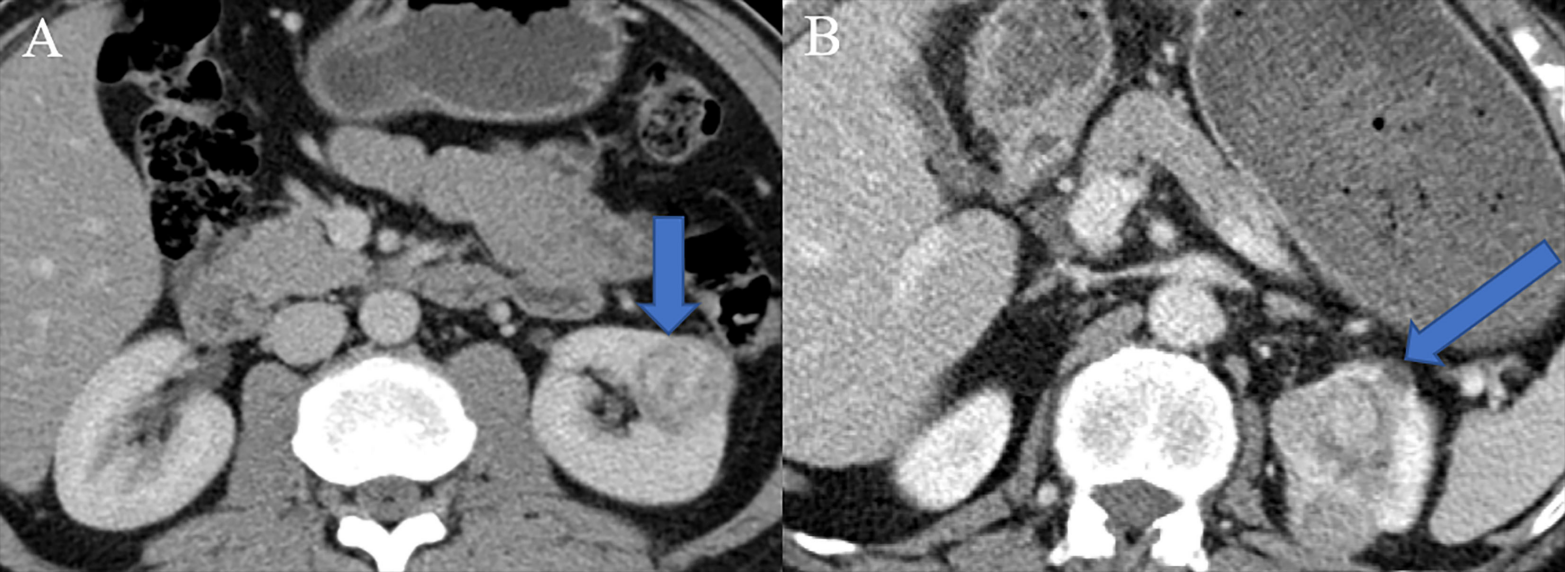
Two representative nephrographic phase CT images. (A) A patient with low-grade CCRCC, there was no specific radiological features in the presented CT image. (B) A patient with high-grade CCRCC, tumor necrosis, angiogenesis, and perinephric invasion phenomena were observed in the presented CT image.
